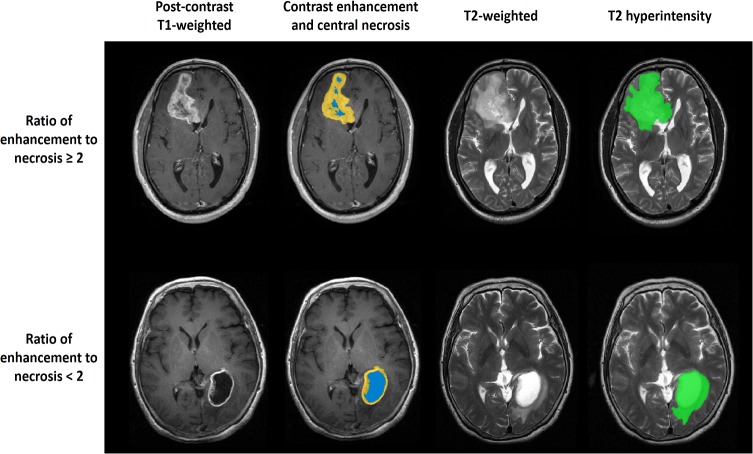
Anatomic MR images for anaplastic gliomas with different ratios of enhancement to necrosis (≥ 2 and < 2). The areas of contrast enhancement are marked in yellow; areas of central necrosis are marked in blue; areas of T2 hyperintensity (exclusion of contrast enhancement) are marked in green.
